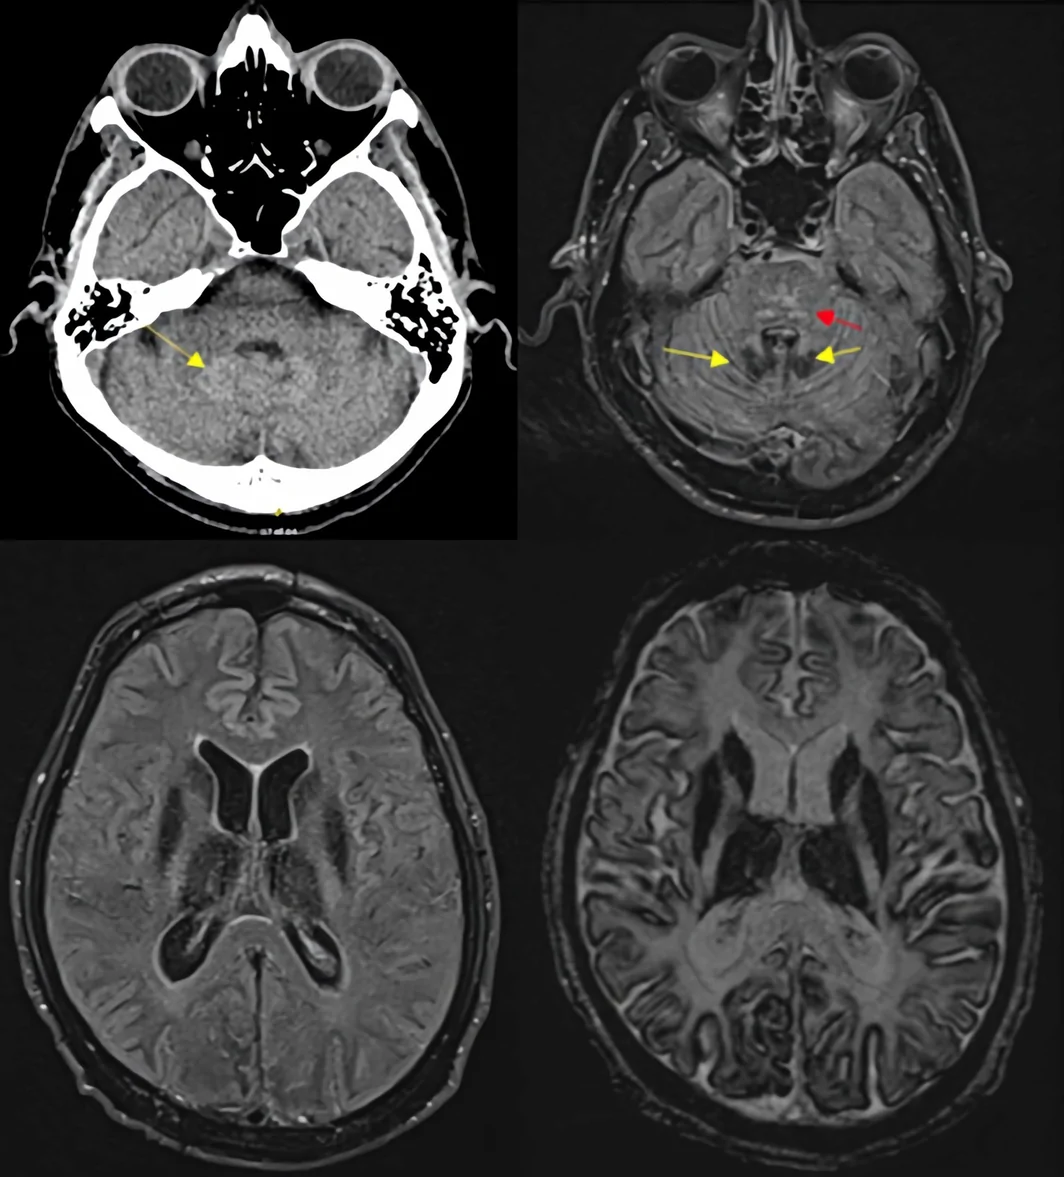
CT and MRI of the brain showed iron deposition in basal ganglia and dentate nuclei. CT brain (axial) showing a hyperdense signal (yellow arrow) in the dentate nuclei (top left). MRI brain FLAIR sequence showing a white matter hyperintensity along the corticospinal pathway extending to the pons (red arrow) and a hypointense signal in the dentate nuclei (yellow arrows) (top right). MRI brain Fluid Attenuated Inversion Recovery (FLAIR) shows hypointense signals in the bilateral thalami, caudate, and lentiform nuclei, as well as a hyperintense signal in the posterior limb of the internal capsule (bottom left). MRI brain SWI confirming iron deposits (bottom right).
